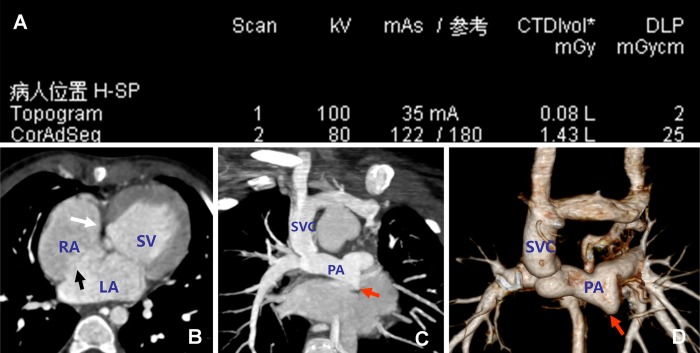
Representative multi-slice spiral computed tomography images from a male patient aged 3 years. A. Scanning parameters. CTDIvol, volume computed tomography dose index; DLP, dose length product (DLP). The effective radiation dose was calculated to be 0.018×2.3×25 = 1.035 mSv. B. Multiplanar reconstructed image showing a single ventricle, tricuspid valve atresia (white arrow), and a large atrial septal defect (black arrow). C. Maximum intensity projection showing the connection between the superior vena cava and right pulmonary artery (after surgery with the Glenn procedure), and primary pulmonary artery atresia (red arrow). D. Volume rendered image showing the features seen in C. LA, left atrium; PA, pulmonary artery; RA, right atrium; SV, single ventricle; SVC, superior vena cava.
